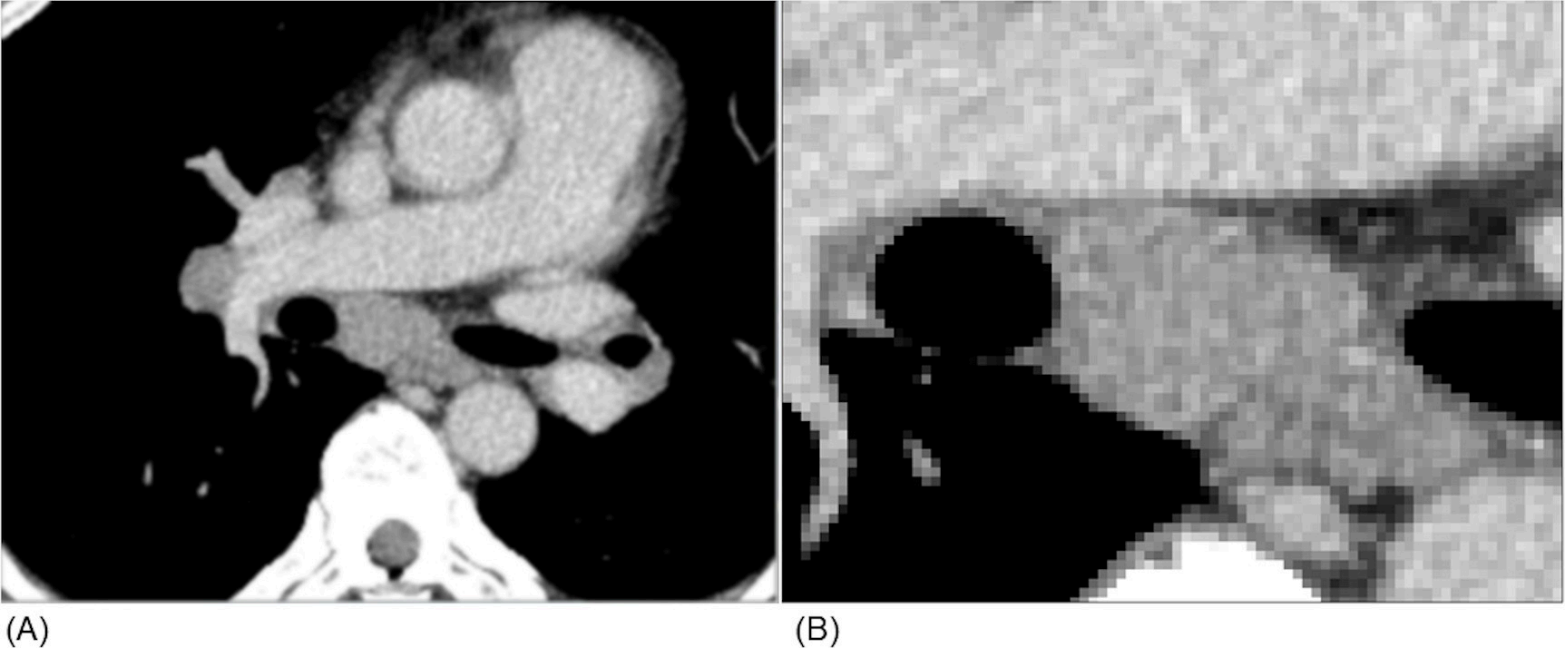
CT image of enlarged lymph nodes due to sarcoidosis. An enlarged mediastinal lymph node, as well as slightly enlarged hilar nodes, are shown on an enhanced CT image (A). The density of the mediastinal lymph node seems basically homogeneous (B); however, distinguishing enlargement due to sarcoidosis from that due to lymph node metastasis from small cell lung cancer on CT images only is difficult.
